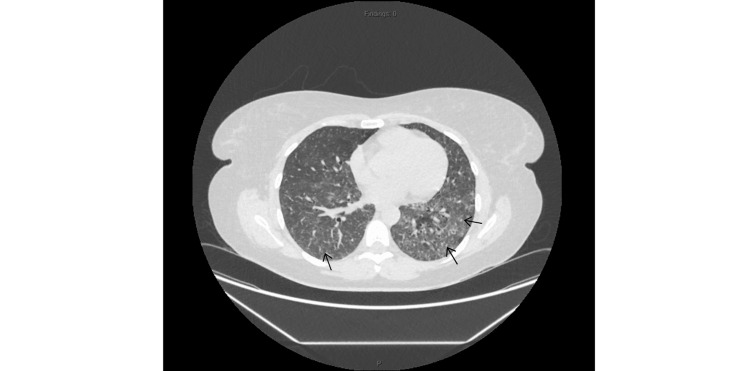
High-resolution computed tomography (HRCT) chest demonstrating diffuse bilateral ground-glass opacities and basal interstitial thickening (arrows), features suggestive of interstitial lung disease, likely nonspecific interstitial pneumonia (NSIP) type. High-resolution CT chest showing progressive multifocal bilateral ground-glass opacities in both lungs, most predominantly in the lower lobes, with associated mild bronchiectasis and interstitial thickening,but without honeycombing.
